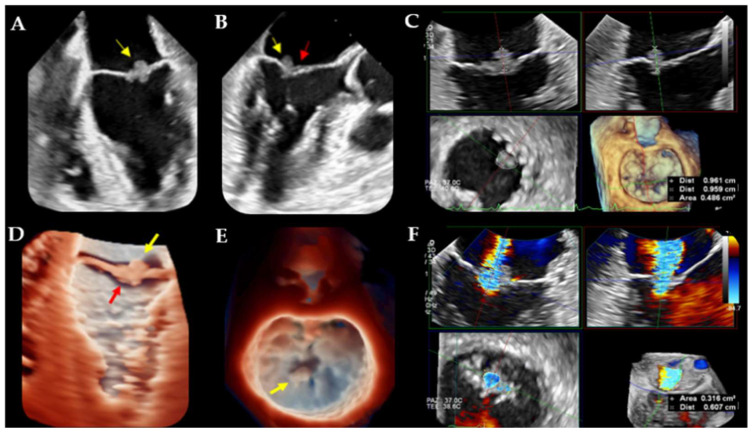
(A,B) Mid-esophageal transesophageal echocardiogram (TEE) views showing an iso-echogenic mass on P2 scallop tip (yellow narrow) and a thickened A2 edge (red narrow). (C) Three-dimensional TEE multiplanar reconstruction focused on P2 lesion size: 9.6 mm in length, area of 48 mm^2^. (D,E) Three-dimensional TEE-true view technique—a live 3D TEE (E) and a 3D “zoom” surgical view—(F) showing mitral valve lesions on both atrial (yellow narrows) and ventricular sides (red narrow). (F) A moderate mitral valve regurgitation in a 3D TEE multiplanar reconstruction: Proximal Isovelocity Surface Area (PISA) radius = 6 mm, VCA (vena contracta area) 3D = 0.3 cm^2^.
